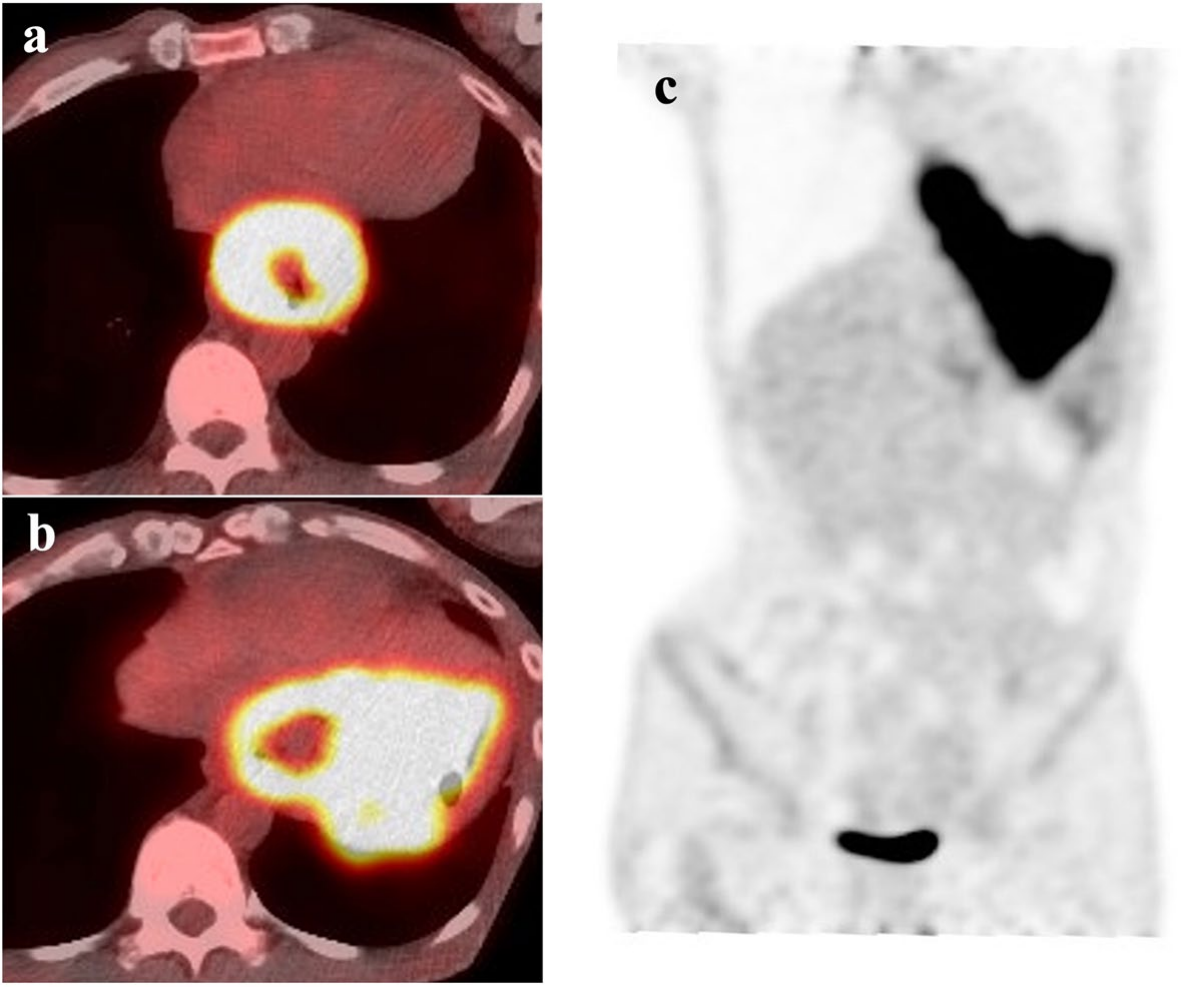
PET-CT findings. a and b Initial PET-CT showed hypermetabolism of esophageal tumor (SUVmax = 13.355). c No metastases to distant organs were detected
